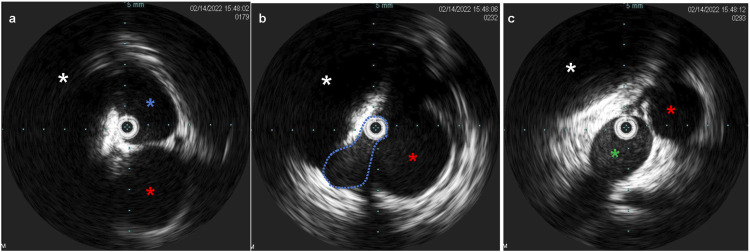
(A) Intravascular ultrasound (IVUS) demonstrates a patent infrarenal left IVC (blue asterisk) adjacent to the aorta (red asterisk). (B) IVUS at the retroaortic IVC/renal vein segment demonstrates compression of the venous lumen (outline in blue) behind the aorta (red asterisk) against the spine (white asterisk). (C) Above the retroaortic segment, the IVC continues as the patent enlarged azygos vein (green asterisk), adjacent to aorta (red asterisk) within the thorax.
